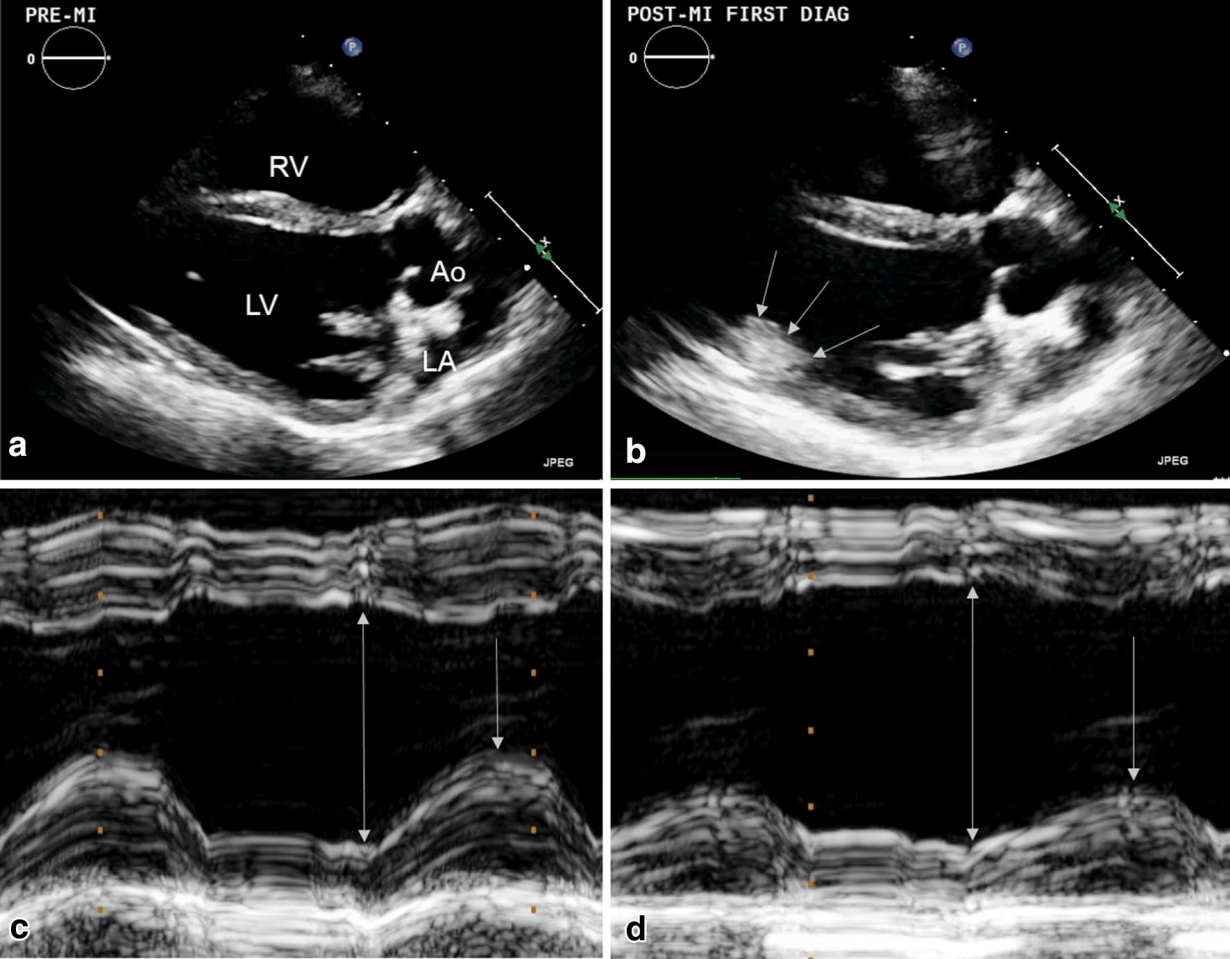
Two-dimensional (2D) and M-mode echocardiography prior to and following D1-LAD occlusion. Echocardiography was performed prior to (a) and following D1-LAD occlusion. Occasionally, the presence of the infarct could be seen as a hyperechoic amorphous mass on 2D images, associated with the left ventricular posterior wall and anterolateral papillary muscle, shown here outlined by *arrows* (b). Compared to pre-MI M-mode images (c), there was an appreciable decrease in systolic excursion of the left ventricular posterior wall following D1-LAD occlusion (d). The *double arrows* represent the left ventricle at its maximal diastolic diameter, while the *single arrows* represent the maximal systolic excursion of the LV posterior wall.
